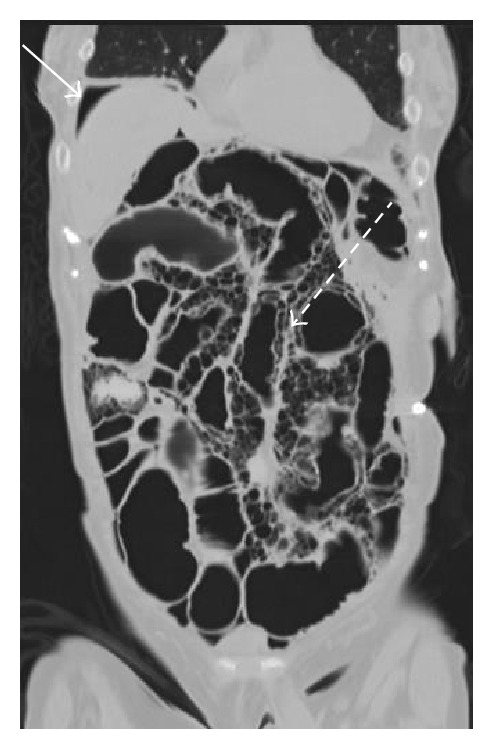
Coronal CT image demonstrates pnemo periotneum in the right upper quadrant of the abdomen, above the liver (arrow). Multiple large “cysts” are seen within multiple loops of small bowel (dashed arrows), consistent with “pneumatosis cystoides intestinalis” seen in this patient with scleroderma.
